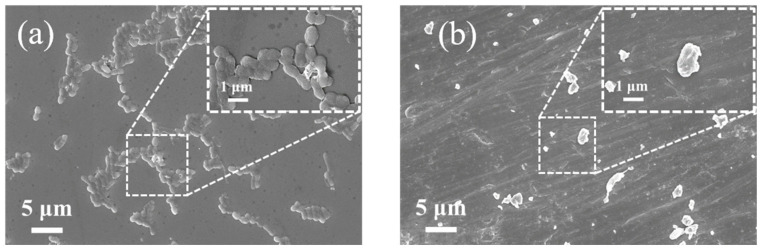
SEM images of *Pseudoalteromonas*. (a) SEM image of *Pseudoalteromonas* before ultrasonic treatment; (b) SEM image of *Pseudoalteromonas* after ultrasonic treatment.
